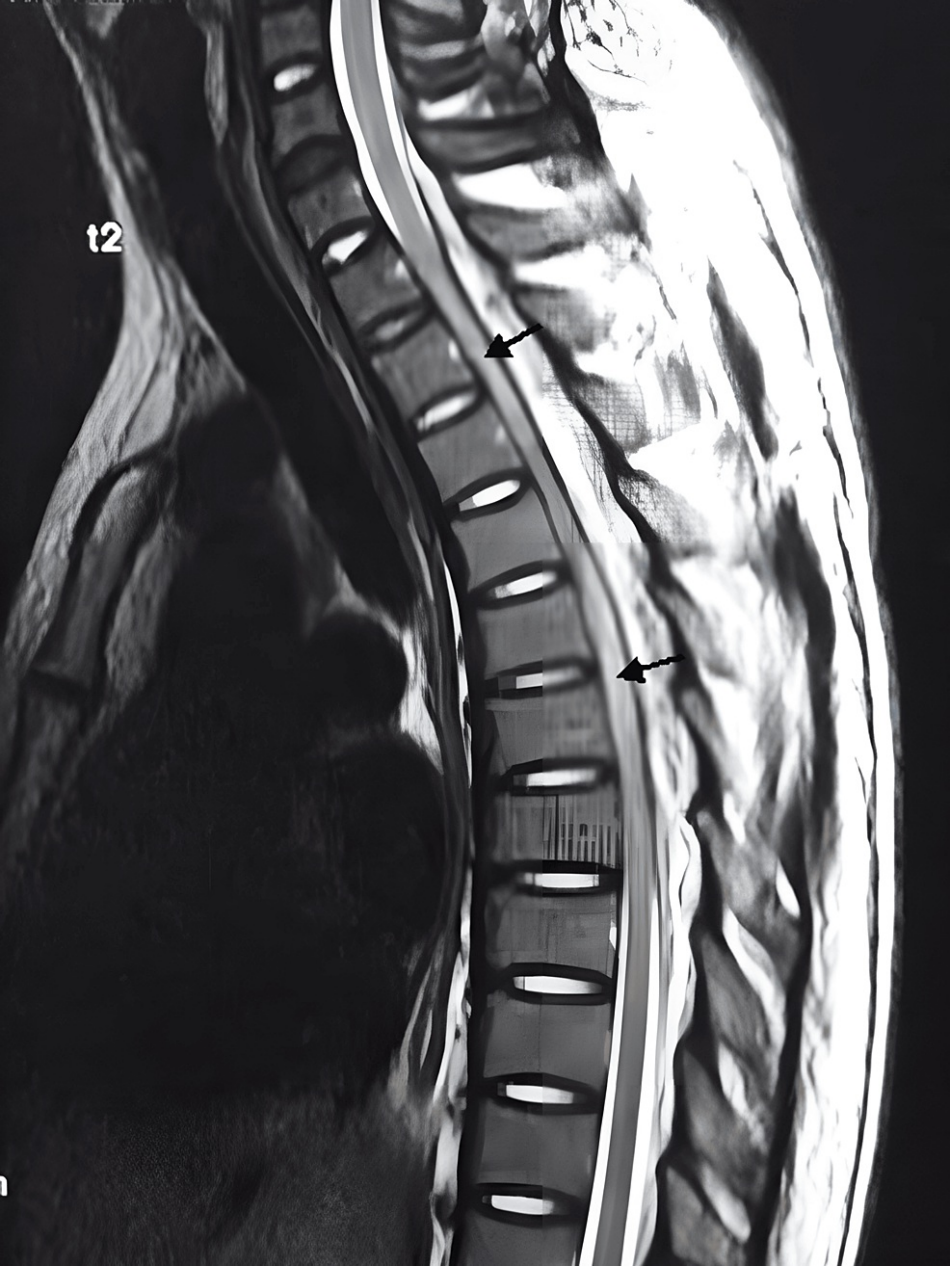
Sagittal T2-weighted MRI The image showing a cervicothoracic spinal epidural abscess at C6-T10 and hyperintense intramedullary images corresponding to areas of myelitis MRI: magnetic resonance imaging
